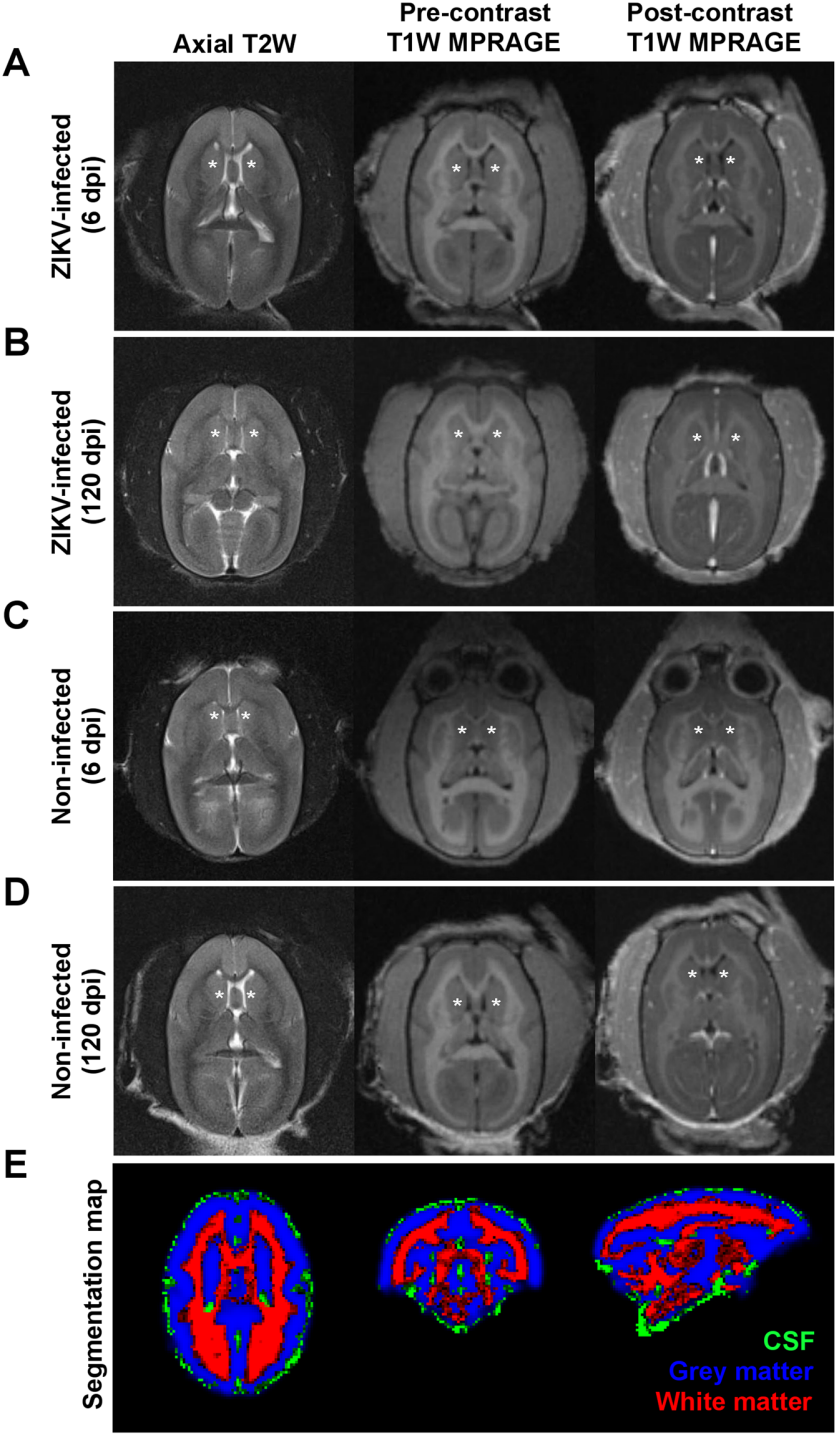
MRI brain images. (A–D) From left to right columns, axial T2-weighted fast spin-echo, pre- and post-contrast T1-weighted MPRAGE images of the marmoset brain at the level of the basal ganglia with asterisk (*) denoting the caudate nucleus. These represent (A,B) ZIKV-infected or (C,D) non-infected marmosets obtained at (A,C) 6 or (B,D) 120 dpi. No focal abnormality or pathological enhancement is seen in the ZIKV-infected brain. (E) Segmentation map of the marmoset brain for white, grey matter and cerebrospinal fluid (CSF).
